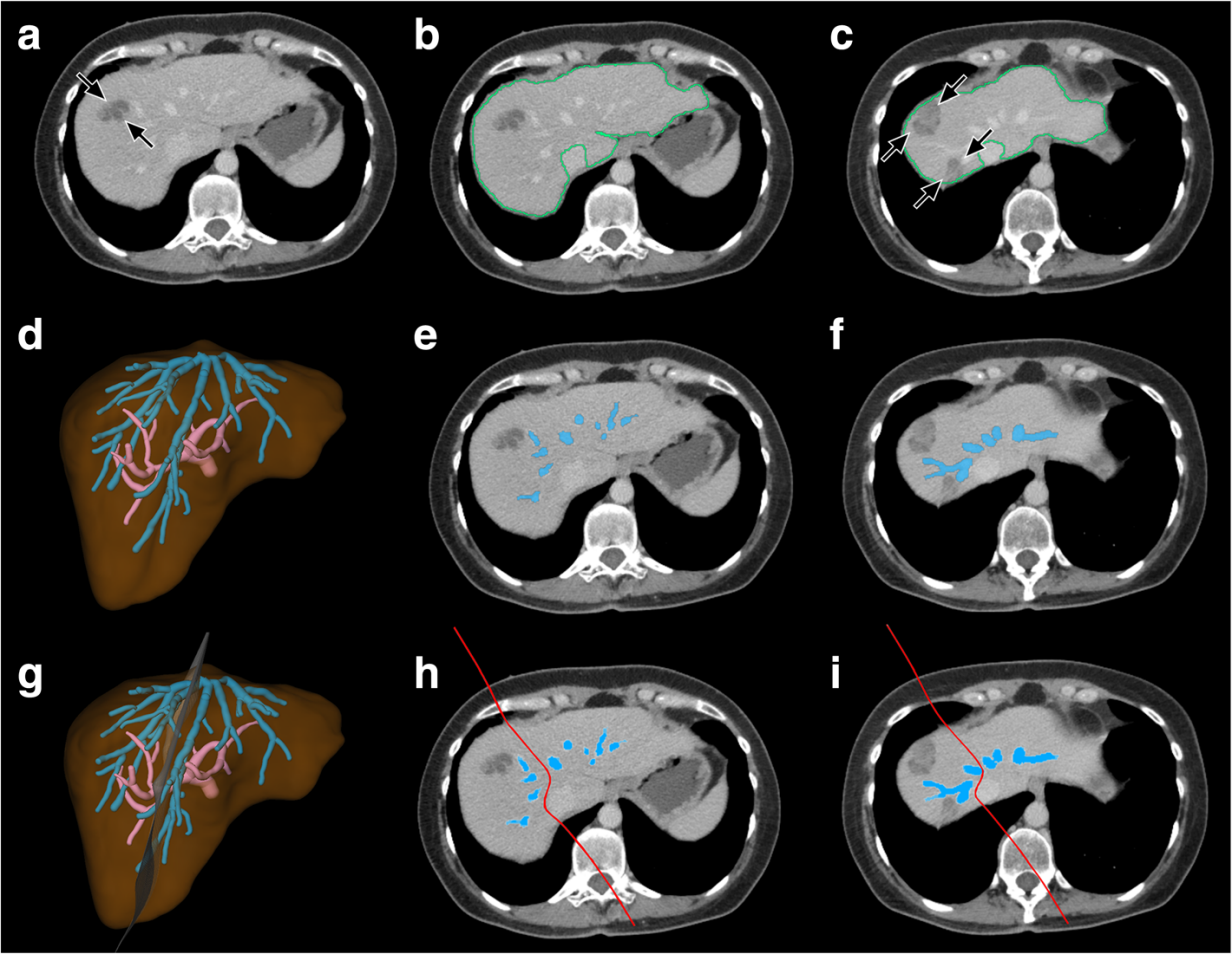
Segmentation process in a patient planned for right hemihepatectomy without resection of the MHV due to metastases of GCT a) Plain axial CT image shows a hypoattenuated metastasis in liver segment 8 (*black arrows*). b) and c) Detection of the liver outline. Further metastases are depicted at the level of the proximal MHV in c) (*black arrows*). d) – f) Detection of the intrahepatic PV (*in pink*) and HV (*in blue*). g) – h) Definition of the transection plane (*in red*)
